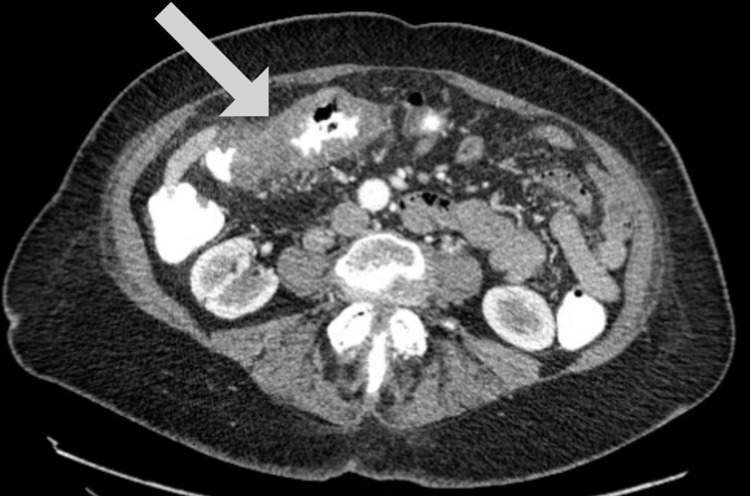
CT abdomen and pelvis showing mural thickening of transverse colon at the hepatic flexure with narrow lumen as demarcated by the arrow.
